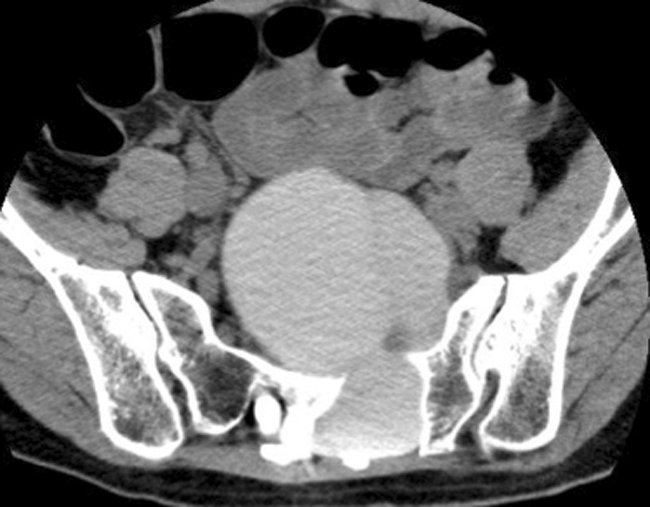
CT myelography in case 10 showing the huge cyst (supine position, caudal view). The left S2 root is compressed and the S3 root is continuous with the cyst interior
